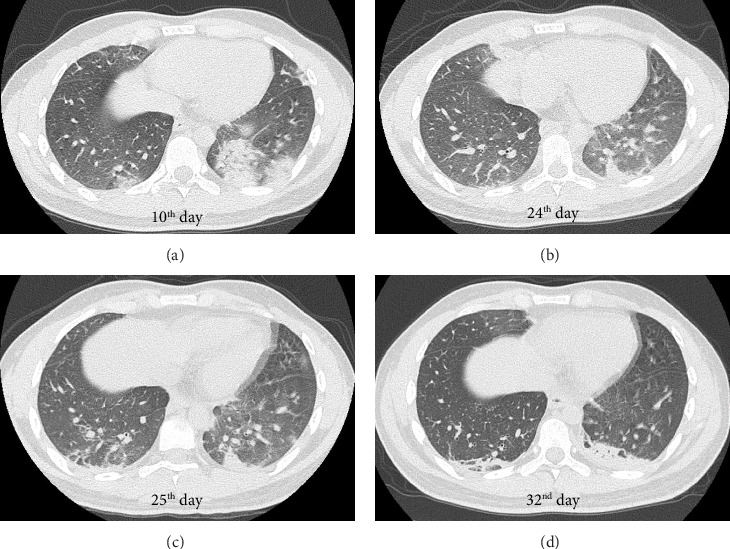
Changes in the chest computed tomography (CT) images. ^∗^The scanning level is different between (a) and (b–d). The level of scanning in (a) is more cranial compared to that in (b–d). ^#^Note that the day of coronavirus disease (COVID-19) onset is counted as the “0^th^ day.” (a) CT image on the day of the first admission (10^th^ day of the COVID-19 onset), showing multiple infiltrative shadows in both lung fields, suggestive of COVID-19 pneumonia with or without secondary bacterial pneumonia. (b) CT image on the day of the second admission (24^th^ day of the COVID-19 onset), showing improvement in bilateral infiltrative shadows. A crescent moon-shaped consolidation remained in the left-lower lung lobe, and ground-glass attenuations newly appeared in both lower lung lobes. (c) CT image on the 2^nd^ day of the second admission (25^th^ day of the COVID-19 onset), showing new linear consolidations on the dorsal side of the right lower lung lobe. (d) CT image on the 9^th^ day of the second admission (32^nd^ day of the COVID-19 onset). Ground-glass attenuations improved, and linear consolidations spread to a plate shape in both lower lung lobes, which was suggestive of organizing changes.
